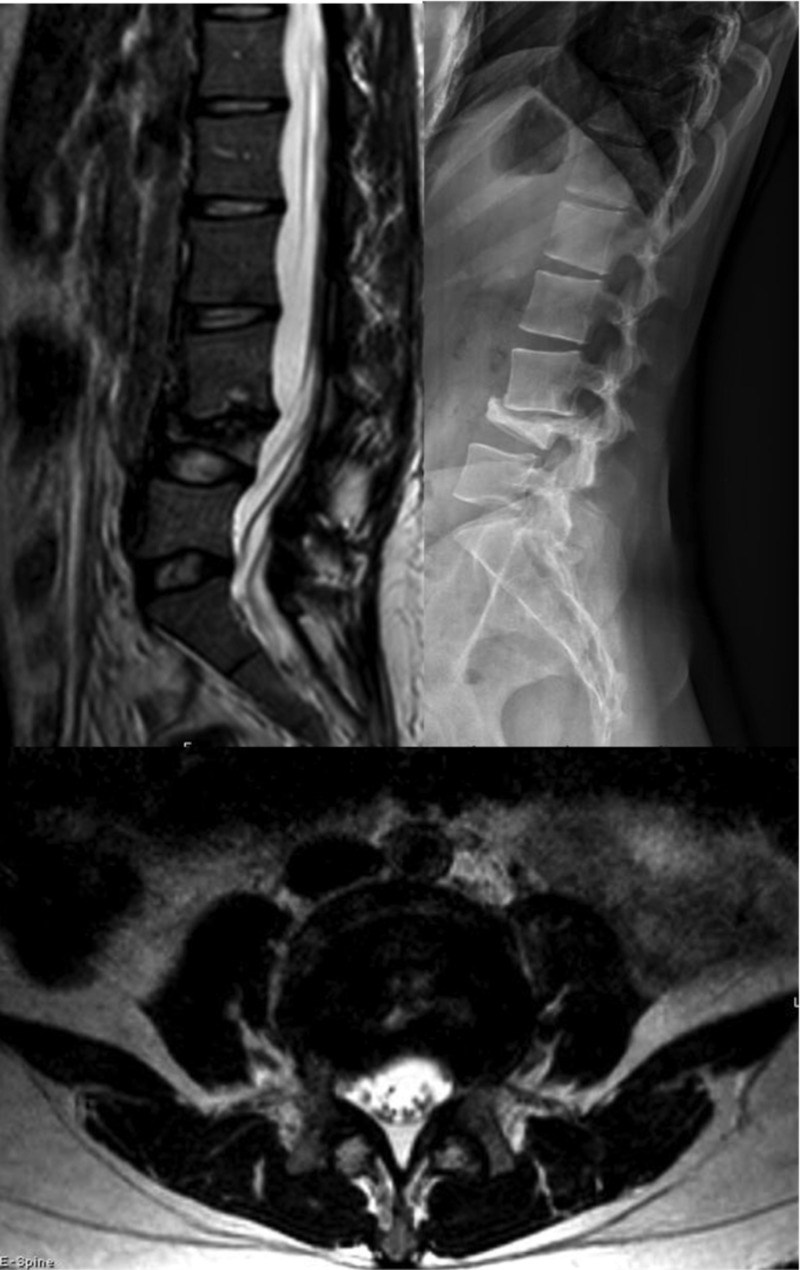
MRI thoracic spine and thoracic spine radiograph Magnetic resonance imaging (MRI) of the thoracic spine T2 sagittal (top left) and axial (bottom) views showed decreased evidence of osteomyelitis and resolution of the psoas collection. A plain radiograph flexion-extension (top right) showed stable loss of vertebral body height and stable grade 1 spondylolisthesis at L3-L4, without any increase in angular deformity or motion.
